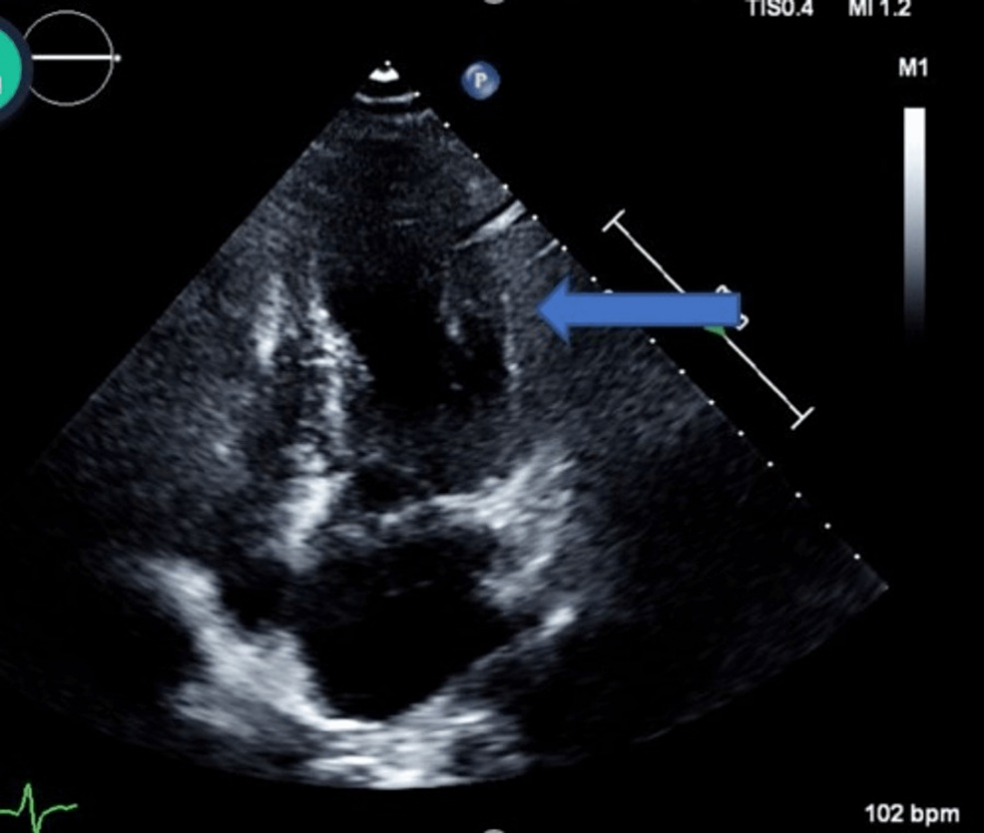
Transthoracic echocardiography showing apical balloning (blue arrow)
